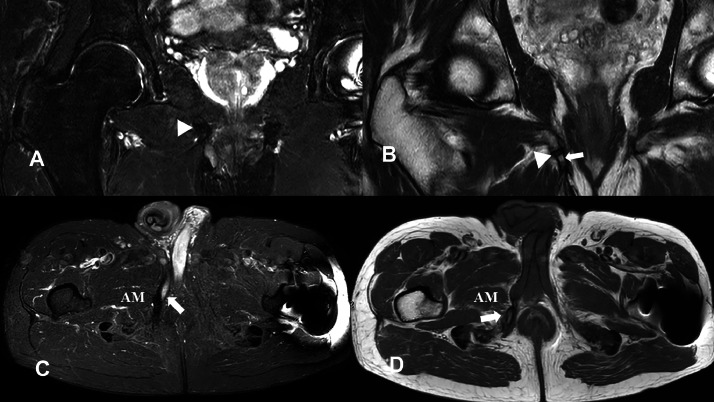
Pelvic MRI (1.5 Tesla). (A) and (B) Coronal T2 Dixon and T1-weighted images showing a pelvic digit with high T1 and low T2 signal intensity, consistent with cortico-medullary bone components (white arrow), and a proximal pseudoarthrotic joint with the ischiopubic branch (white arrowhead). (C) Axial STIR image demonstrating the extension of the digit towards the hypertrophied insertion of the right corpus cavernosum (white arrow). (D) Axial T1-weighted image showing direct contact with the insertion of the right adductor magnus (AM) muscle (white arrow).
